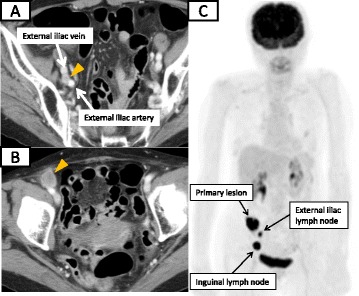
CT shows the enlarged right external iliac (a) and inguinal (b) lymph nodes, and FDG-PET shows a significant accumulation of FDG into the primary colon lesion with the SUV of 17.9 and external iliac and inguinal lymph nodes with SUV of 9.1 and 25.1, respectively (c). The *yellow arrowheads* indicate lymph node
